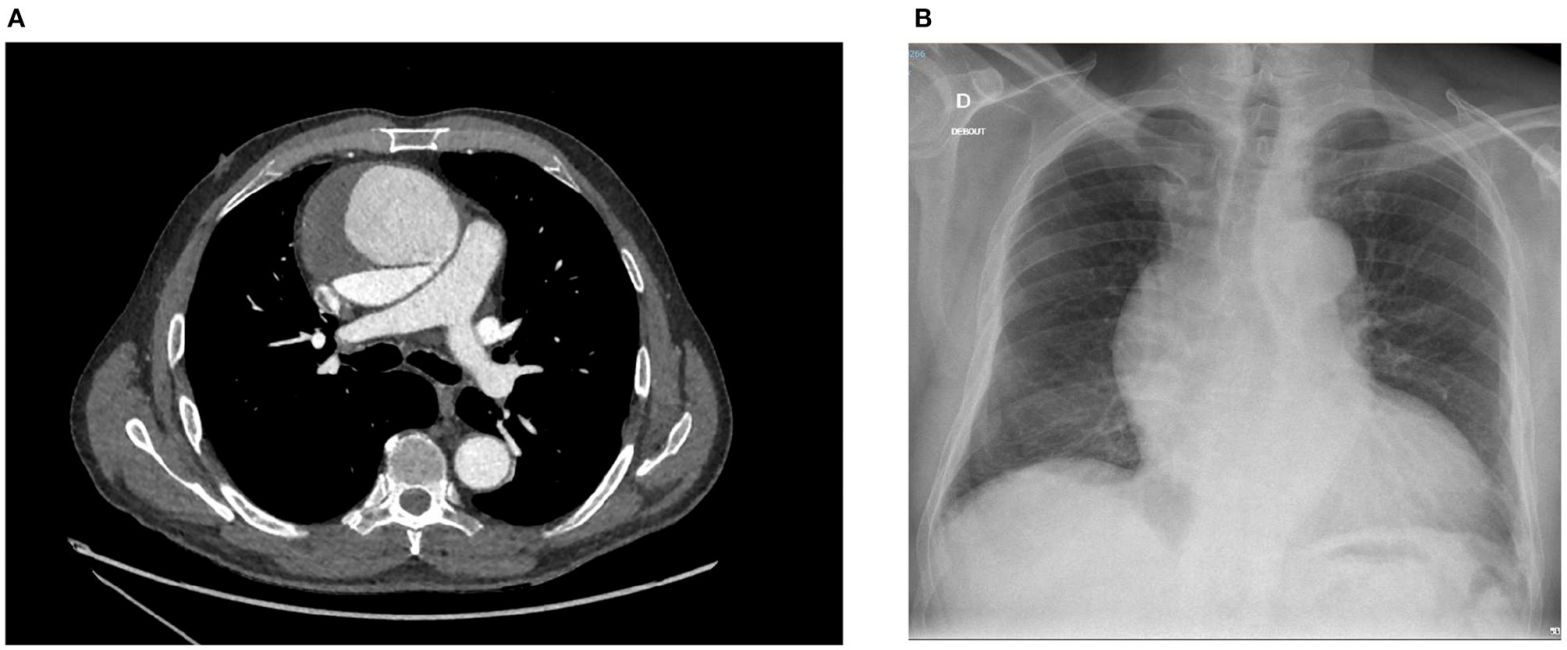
(A) Contrast medium thoracic CT scan showing a CTAAD with a thick intimal tear (7 mm) and an enlarged ascending aorta (82 × 87 mm). The false lumen is partially thrombosed. (B) Chest X-ray showing an aortic aneurysm with a widening of the mediastinal silhouette, an enlargement of the aortic knob, and a displacement of the trachea from the midline. CT, computed tomography; CTAAD, chronic type A aortic dissection.
